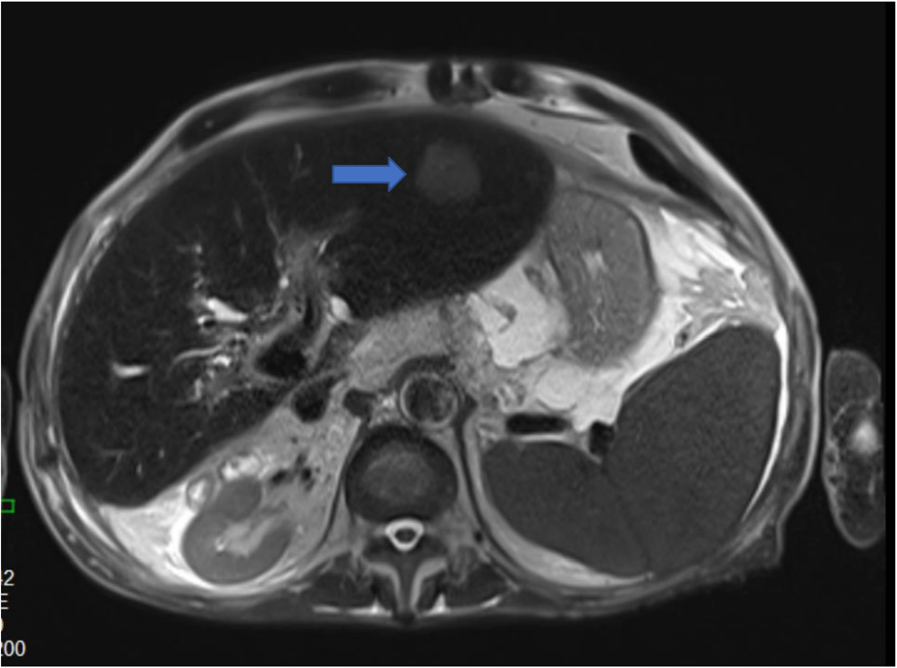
Additional slice of the MRI showing the nodule in S2 (arrow) 12 months after the end of treatment and SVR
